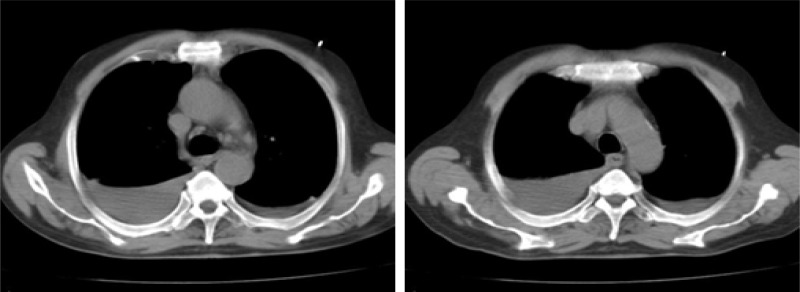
Chest CT scan after 2 weeks indicating the retrosternal mass resolved
